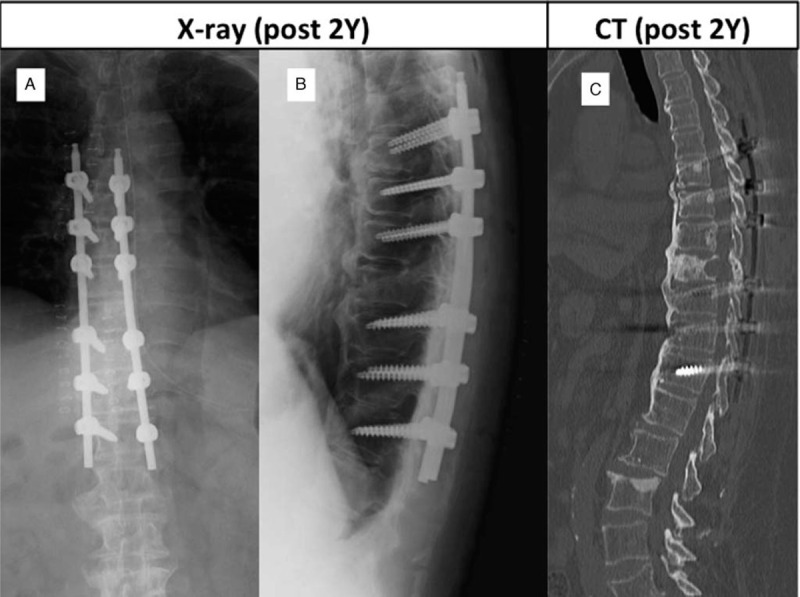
(A, B) Radiographs and (C) computed tomography scan showing ossification and fusion of the site of Metastasis at T8.
